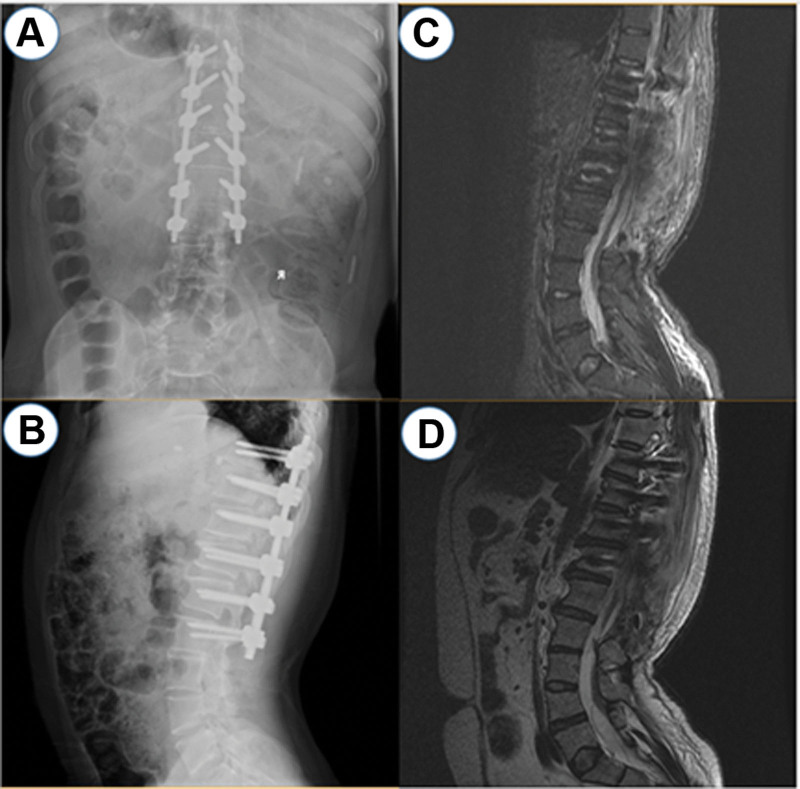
Anteroposterior (A) and lateral (B) plain radiographs of the lumbar spine obtained post-surgery. An improved sagittal profile is observed. T2-weighted sagittal MRI of the lumbar spine also taken post-surgery at the T9–T12 level (C) and of the low conus medullaris at the L4 level (D). MRI = magnetic resonance imaging.
